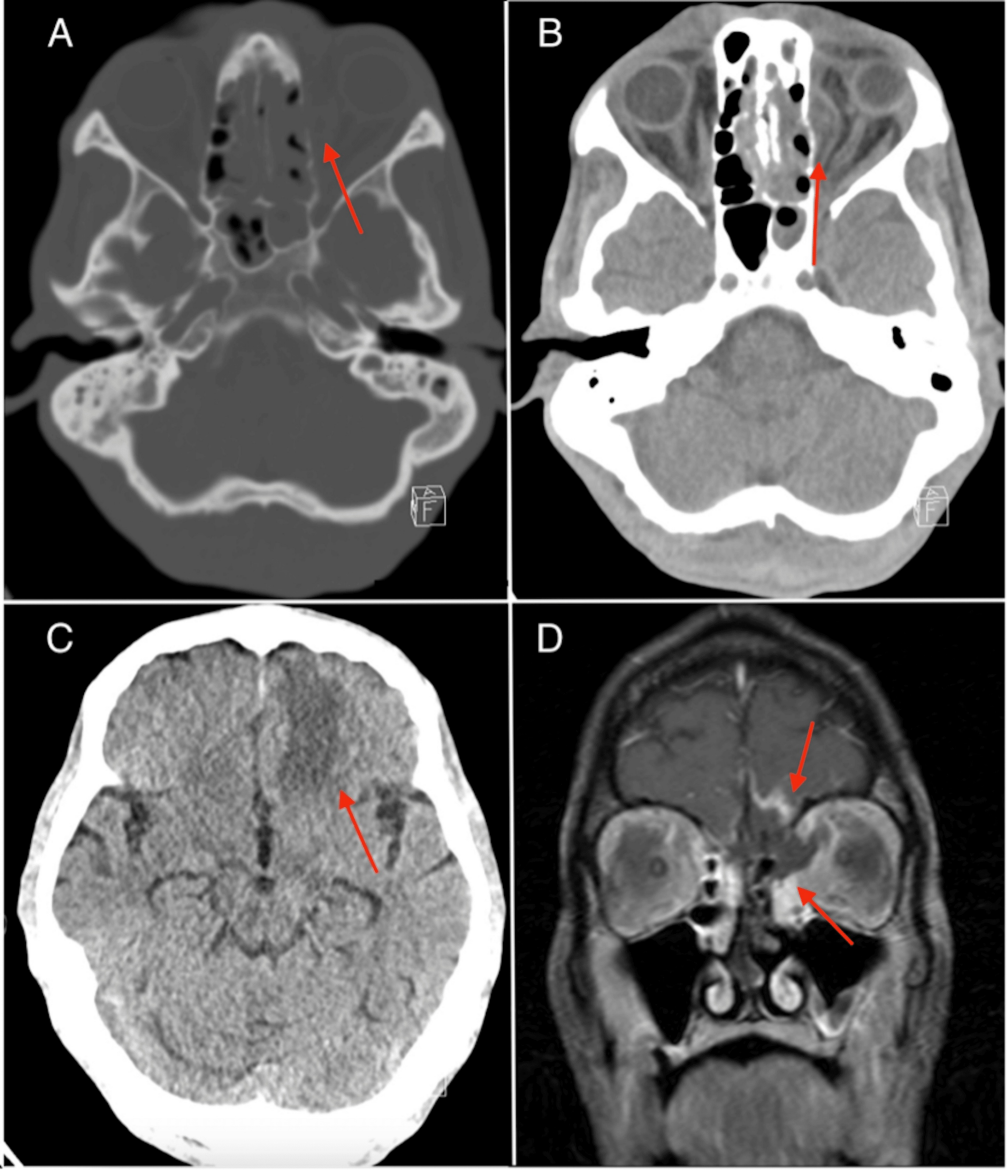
Imaging suggestive of rhino-orbital cerebral mucormycosis Axial non-contrast CT head images show [A] opacification of the ethmoid and sphenoid sinuses with osseous erosion of the left medial orbital wall, [B] trans-osseous extension into the left retro-orbital space, and [C] left greater-than-right frontal lobe parenchymal hypodensity. [d] Coronal T1-weighted fat-saturated contrast-enhanced MRI orbit shows partially non-enhancing sinonasal mucosa with disease extension into the left medial orbit and frontal lobes.
